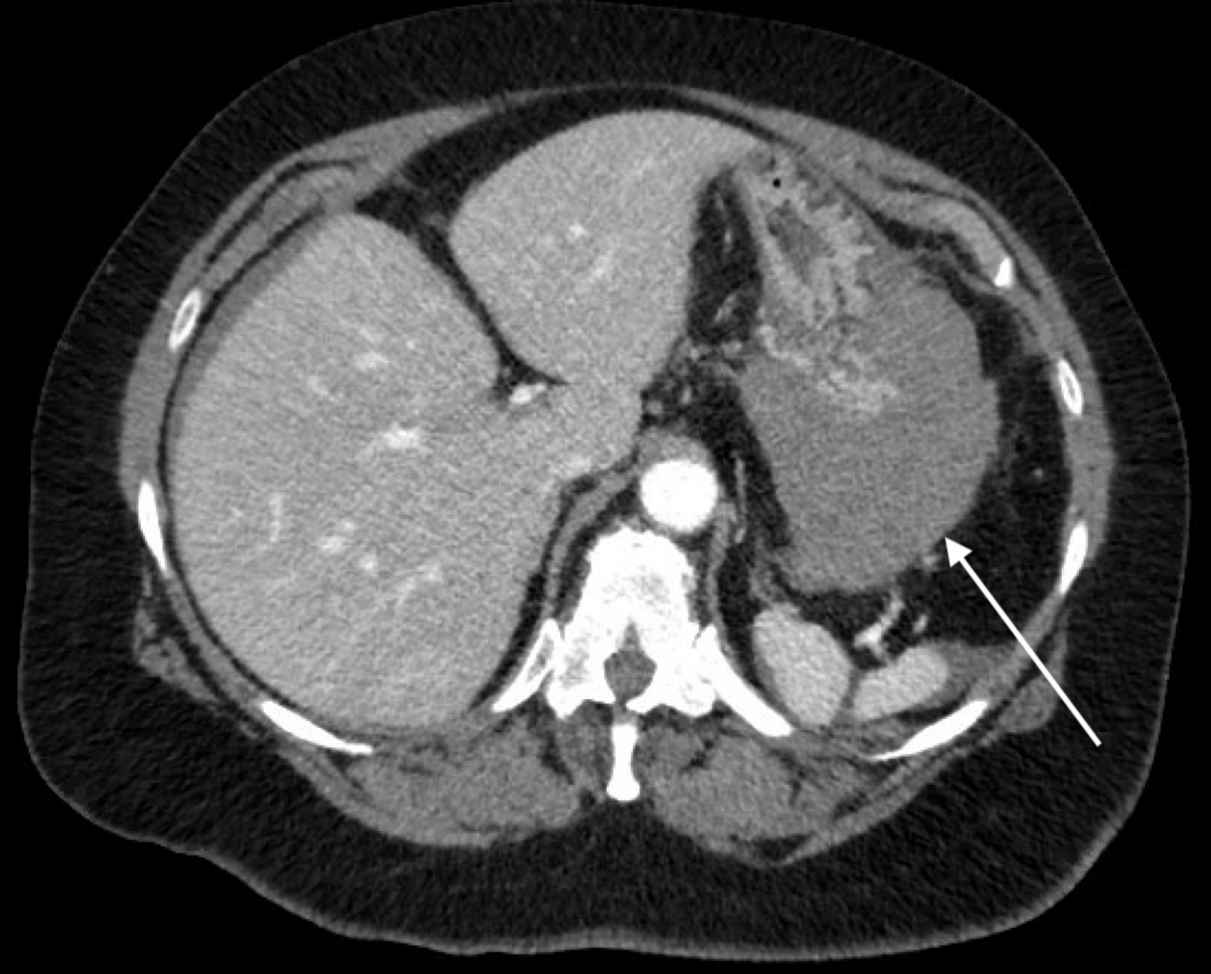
Axial CT image further distal showing high attenuation material adjacent to greater curvature of the stomach (arrow)
